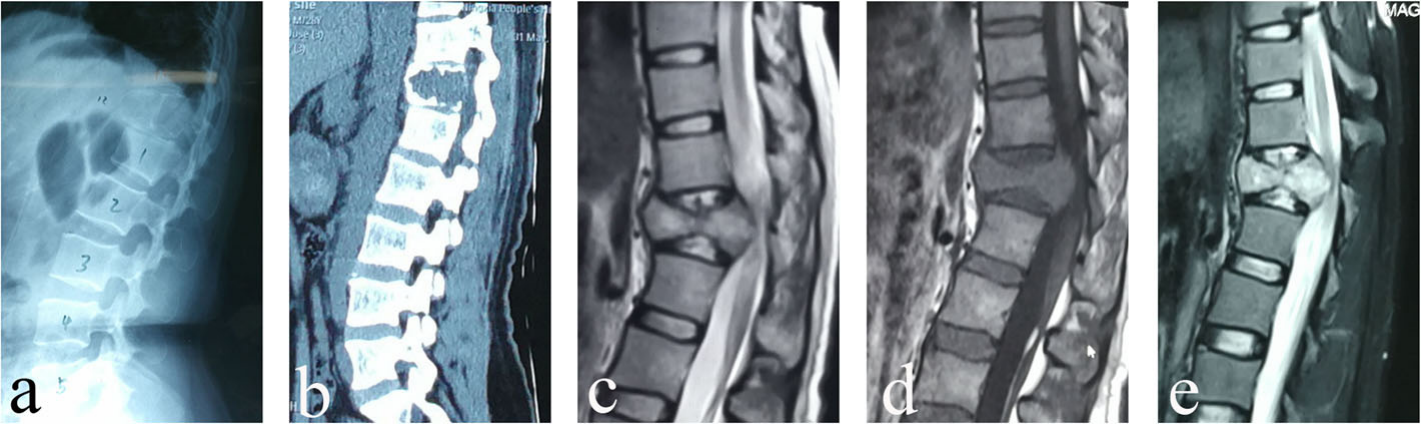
A 28-year-old female patient with a T12 giant cell tumor complicated by neurological deficits. The preoperative sagittal X-ray (a), CT (b), T2-weighted MRI (c), T1-weighted MRI (d), and STIR MRI (e) scans showed a pathological fracture of the T12 vertebral body with spinal cord compression
